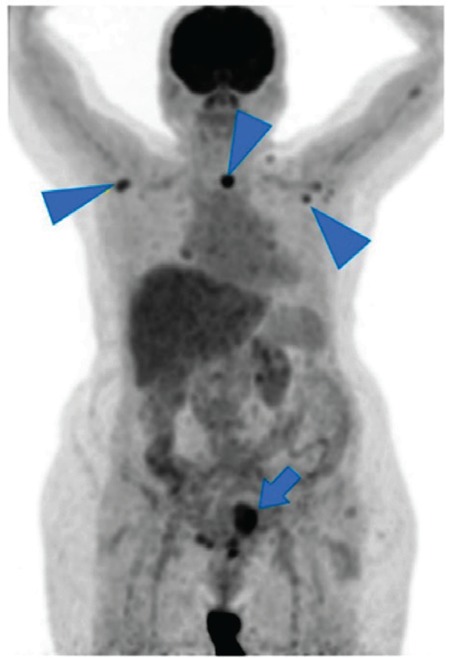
Positron emission tomography/computed tomography maximum intensity projection image shows increased fluoro-D-glucose uptake in uterine cavity (arrow), bilateral axillary lymph nodes and nodular lesion in left thyroid lobe (arrowhead)
